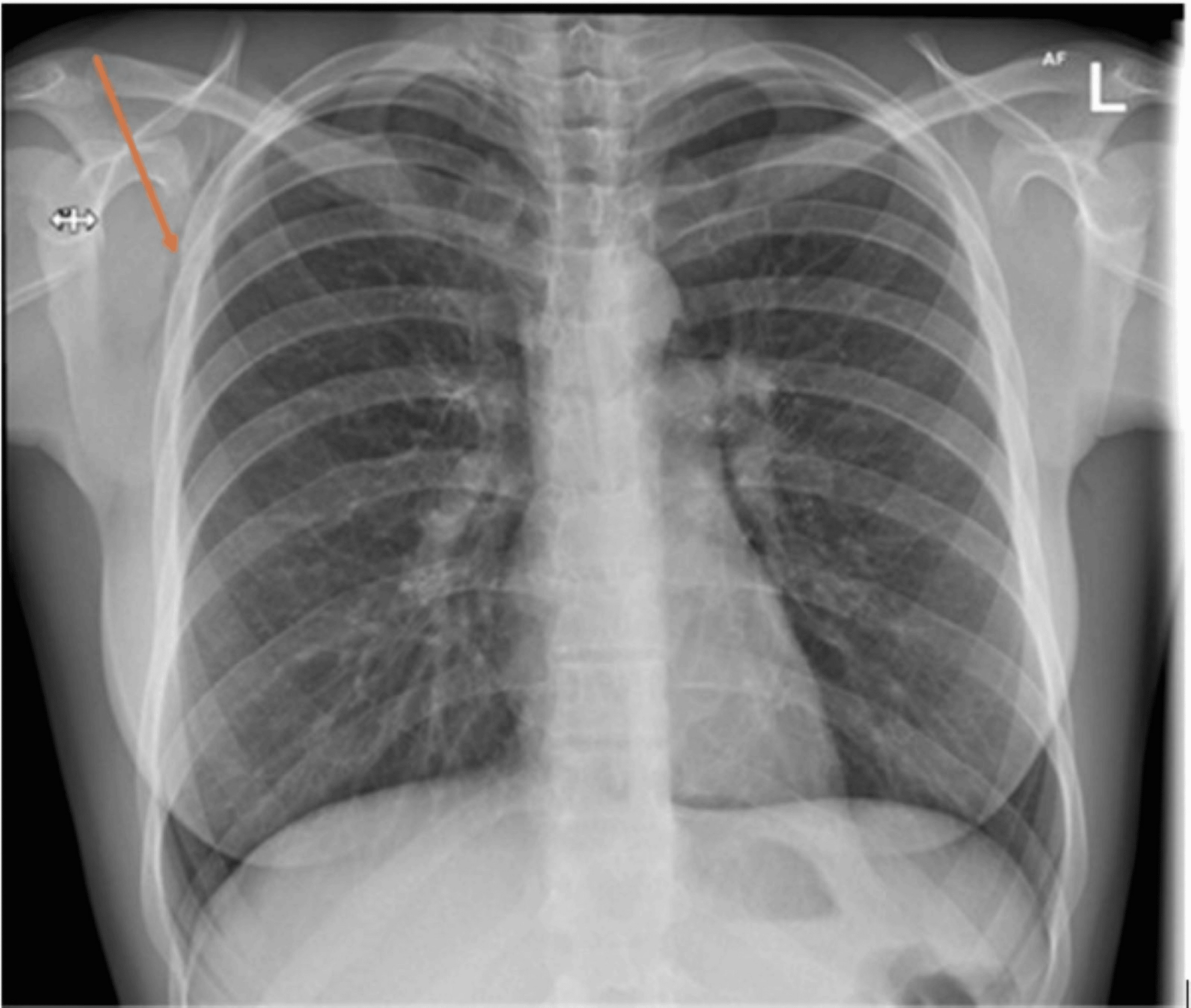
X-ray showing streaking linear lucencies overlying the superior mediastinum and surgical emphysema in the right axilla
